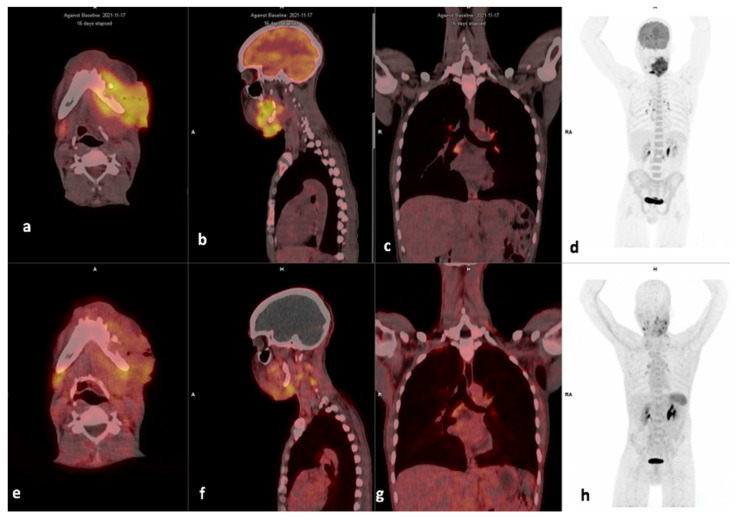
Example of a high FDG, low Pentixafor in mediastinal nodes. Display of maximum intensity projections of [^18^F]F-FDG (d) and [^68^Ga]Ga-Pentixafor (h) and axial and sagittal images of both [^18^F]F-FDG-PET/CT (a,b) and [^68^Ga]Ga-Pentixafor-PET/CT (e,f) (interval between both scans, 16 days) in a 46-year-old HIV-negative female patient oropharyngeal SCC (patient #9). [^68^Ga]Ga-Pentixafor-PET demonstrates mild-moderate inhomogenous tracer accumulation at the site of primary SUVmax 2.63 (a) compared with 16.93 (e) on [^18^F]F-FDG. Multiple foci of intense uptake are seen in inflammatory the mediastinal lymph nodes on [^18^F]F-FDG (c), these appear less intense on [^68^Ga]Ga-Pentixafor-PET (g). Notably, the spleen demonstrates higher uptake on [^68^Ga]Ga-Pentixafor (h) compared to [^18^F]F-FDG-PET/CT (d).
